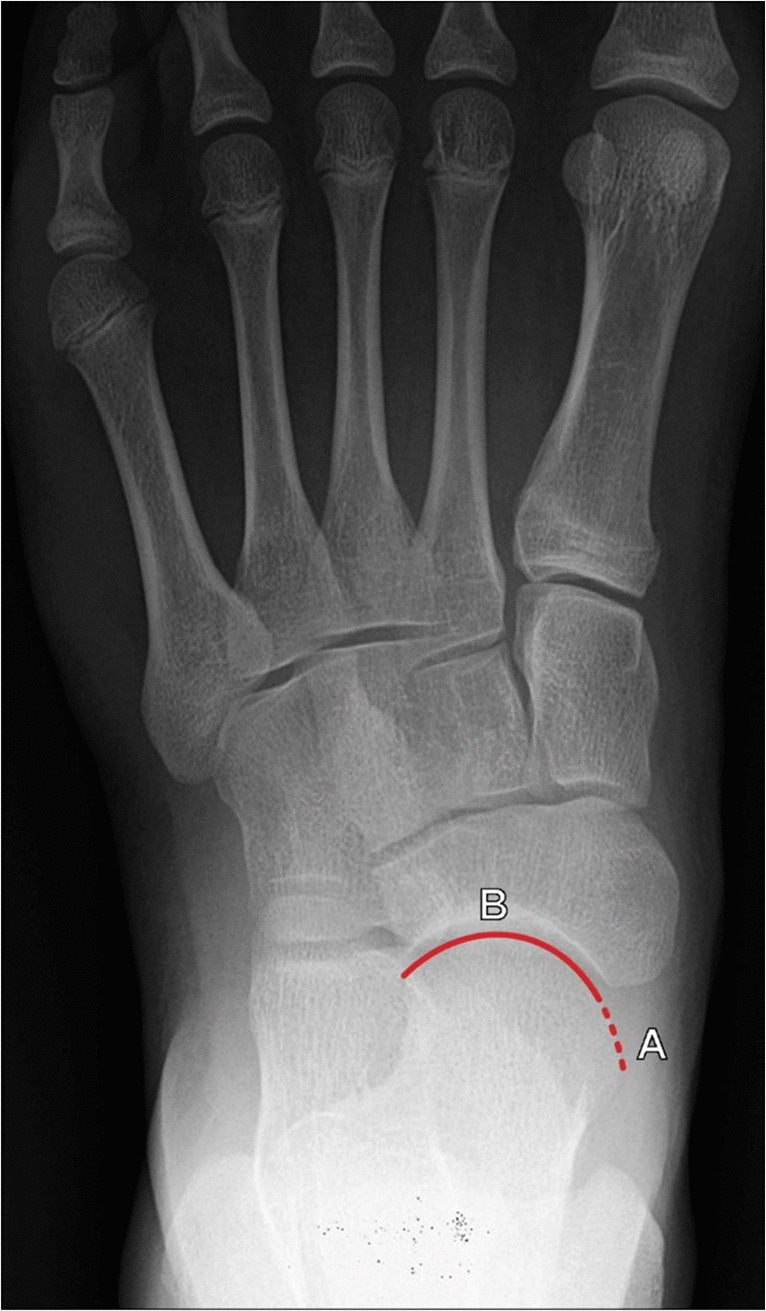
Measurement of the Talonavicular Uncoverage Percentage on DP radiograph of the foot. The portion of the talar head articular surface that is not covered by the navicular (A, dotted line) is divided by the length of the total talar head articular surface (A + B) and multiplied by 100% to give a percentage. A Talonavicular Uncoverage Percentage > 30% is consistent with pathologic flatfoot deformity, and is used to differentiate between stage IIA and stage IIB acquired flatfoot deformity
